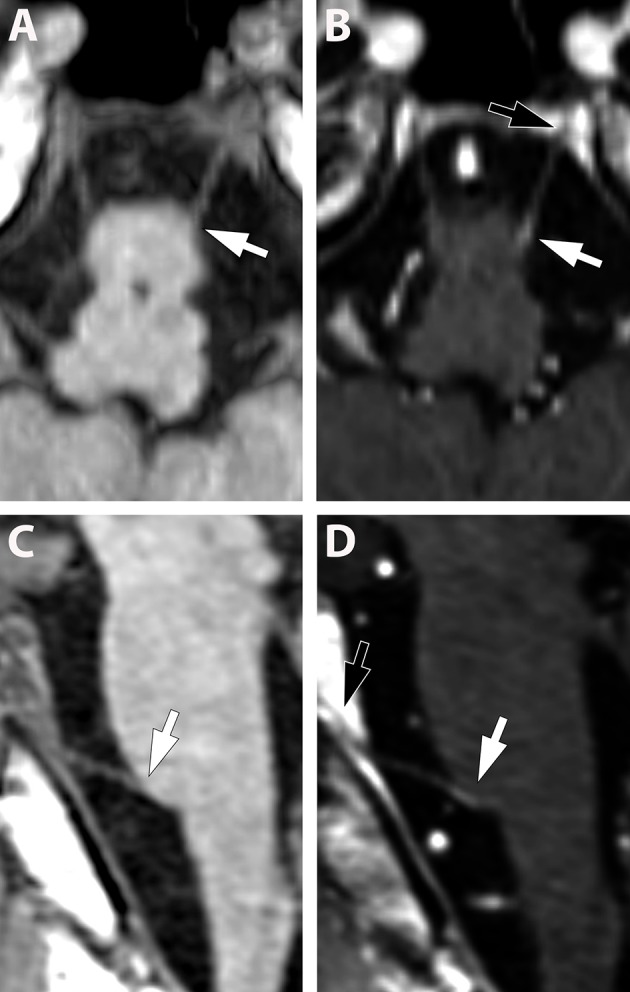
(A–D) (Pat. No. 10): Pre-contrast (A,C) and post contrast enhancement (B,D), axial (A,B), and sagittal (C,D), reconstructed T1-weighted MRIs at the level of the abducens nerve. Due to the small diameter of the cranial nerves, other than the fifth nerve, the contrast enhancement is difficult to detect (white arrows in B,D) and multi-planar reconstructions are helpful. Identification of the abducens nerve and differentiation from blood vessels can be facilitated by visualization of the abducens nerve in Dore'lo's canal (black arrow B,D).
